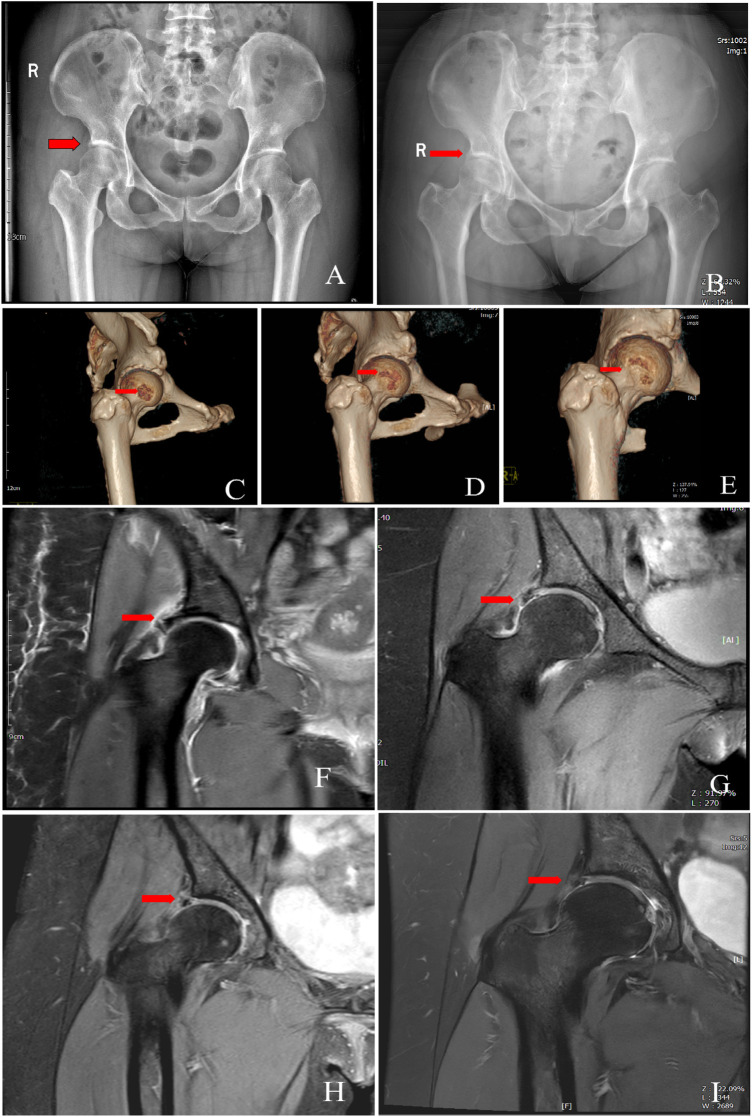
Postoperative radiographs [(A) immediate postoperative, (B) nine months postoperative, anteroposterior view] and the three-dimensional CT [(C) immediate postoperative, (D) six-month postoperative, (E) nine-month postoperative, reconstructed view] demonstrate resolution of the high-density opacity at the anterolateral margin of the right acetabulum and no obvious degenerative changes of the hip joint. (F–I) Postoperative MRI [(F) immediate postoperative, (G) three-month postoperative, (H) six-month postoperative, (I) nine-month postoperative, coronal view] show the repair of the labrum with an autologous gracilis tendon. The nine-month postoperative MRI revealed that the labral morphology had largely returned to normal. Neither appreciable joint effusion nor surrounding soft-tissue edema was observed.
